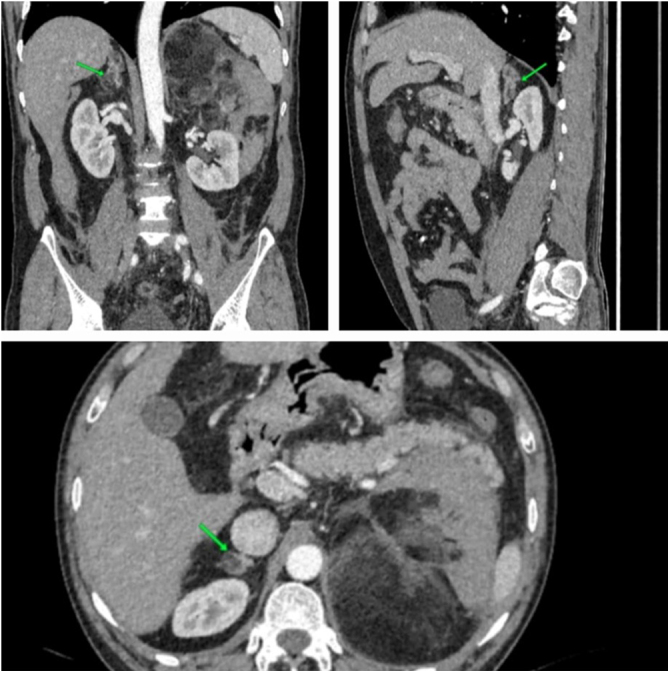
Case 1 – CT Abdomen showing the right adrenal gland with few predominantly fat-density lesions with intermixed soft tissue density suggesting adrenal myelolipoma (yellow arrow) largest measuring 14 mm*12 mm.
